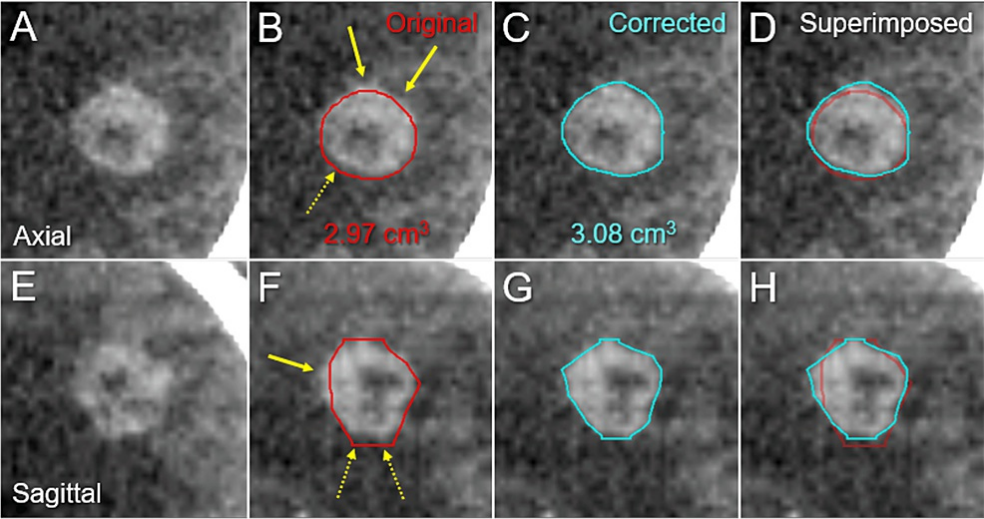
Contrast-enhanced computed tomographic images for stereotactic radiosurgery planning. The images show CECT images (A-H); axial images (A-D); sagittal images (E-H); original contours of the GTV (B, F); corrected contours of the GTV (C, G); and the superimposed images (D, H). (B-D, F-H) The original GTV contours had some under-coverage (arrows in B, F) and over-coverage (dashed arrows in B, F). CECT: contrast-enhanced computed tomography; GTV: gross tumor volume
